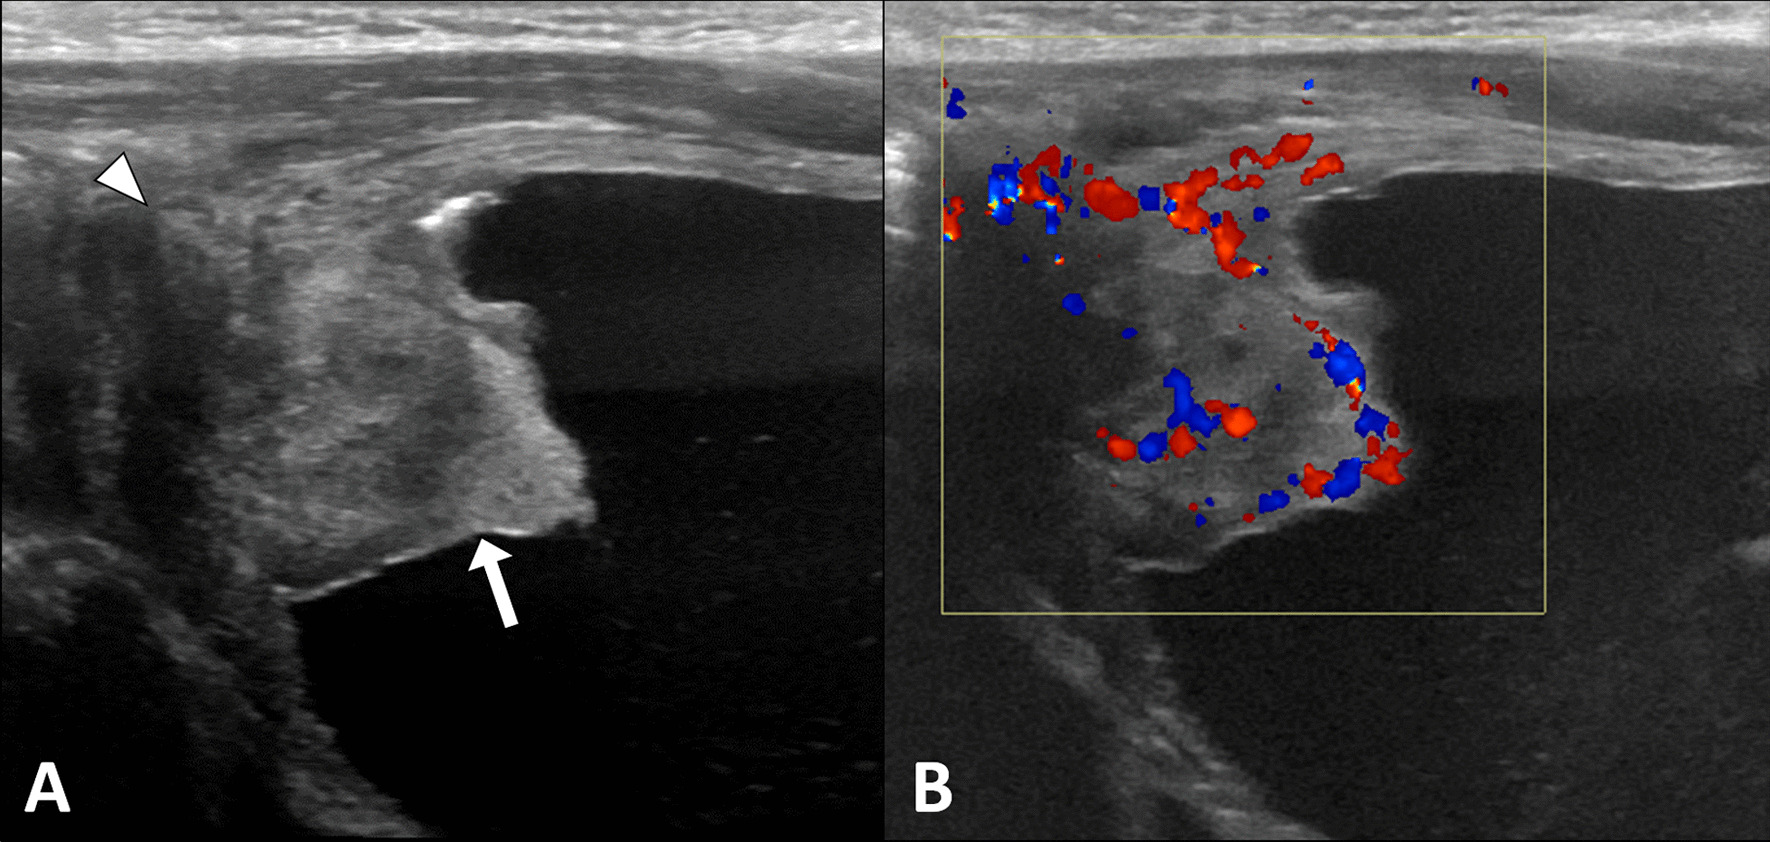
Ultrasound image (A) of the urinary bladder shows a soft tissue lesion (arrow) with an area of suspicious extravesical extension (arrowhead). The color Doppler image (B) shows internal vascularity within the lesion
